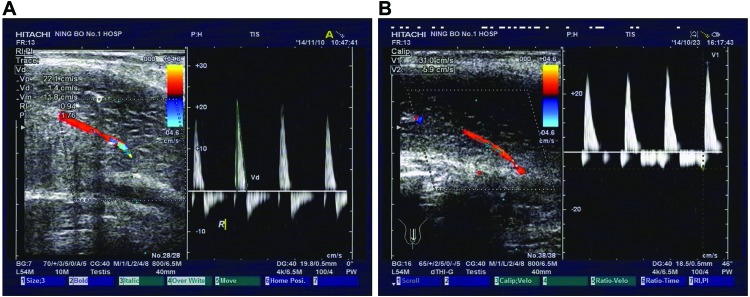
Color duplex Doppler ultrasound scanning of arterial ED patients in the EHS 1/2 (A) and EHS 3/4 (B) groups
